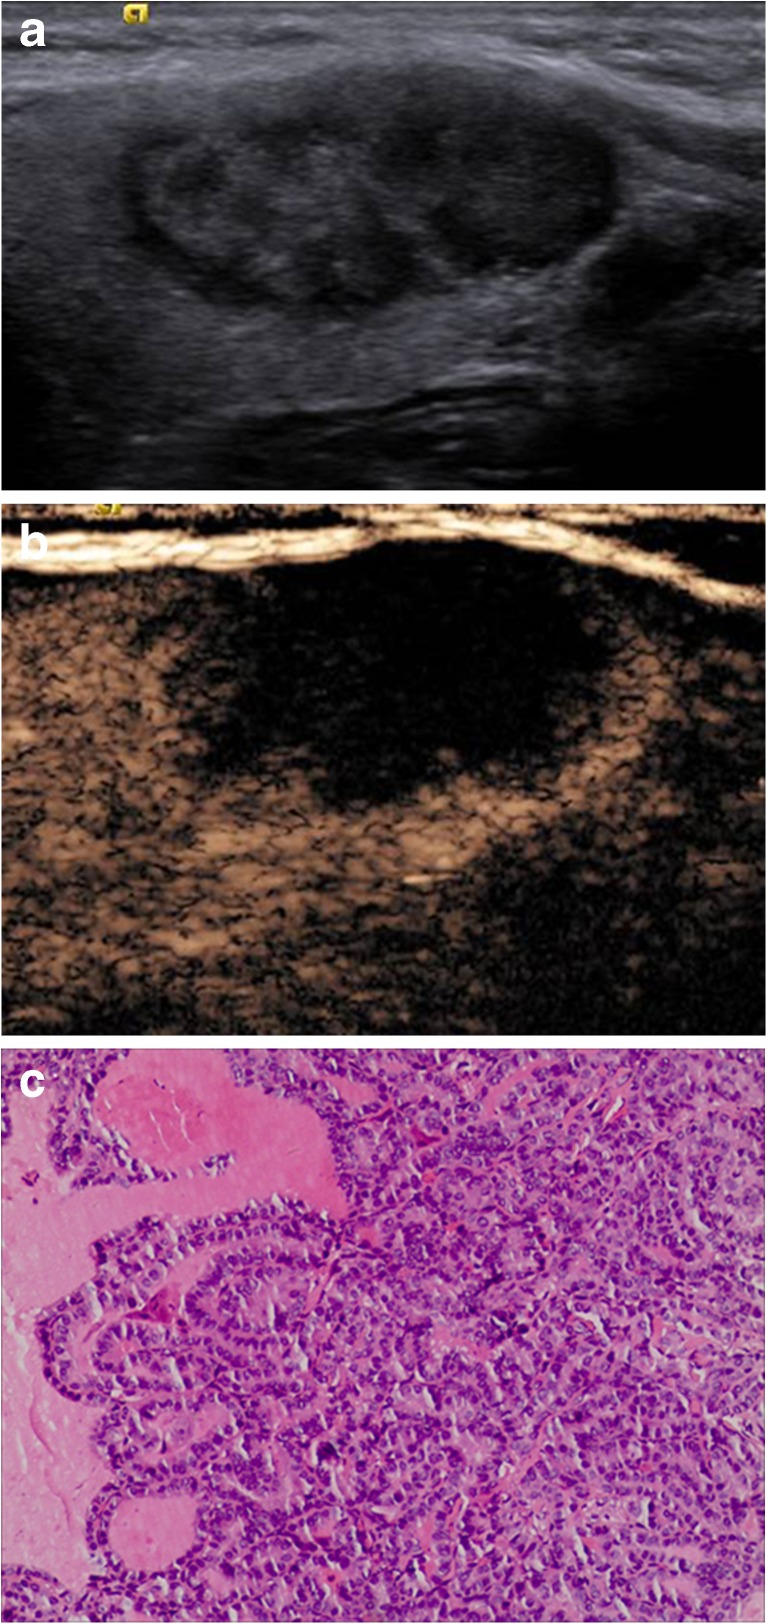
A 42-year-old woman was found to have a 32 × 15-mm solid nodule in the left lobe of her thyroid. a A conventional two-dimensional image. This nodule had two malignant indicators (solid and irregular margin), and it was classified to a 4a TI-RADS score. b An ultrasound contrast image, and the enhancement mode indicates low enhancement. The improved TI-RADS combined with CEUS returned a score of 4b, and the diagnosis indicated a malignant nodule. c The pathological image of the lesion, which is a thyroid papillary carcinoma
